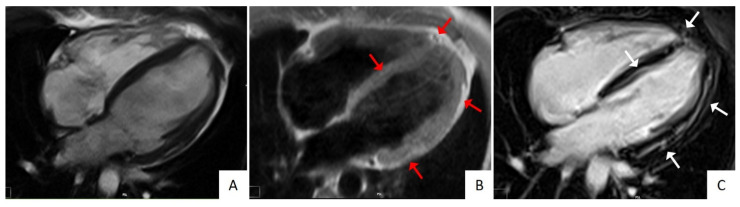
Cardiac magnetic resonance (CMR) imaging features of a patient affected by biventricular arrhythmogenic cardiomyopathy. Cine CMR imaging showing a mildly dilated left ventricle (LV) (Panel A) with a slightly reduced systolic function (not showed). T1-weighted CMR images demonstrating fatty infiltration at the right ventricle apex, lateral, and septal LV walls (Panel B, red arrows). Post-contrast CMR images showing biventricular LGE in the same locations as in Panel B (Panel C, white arrows).
